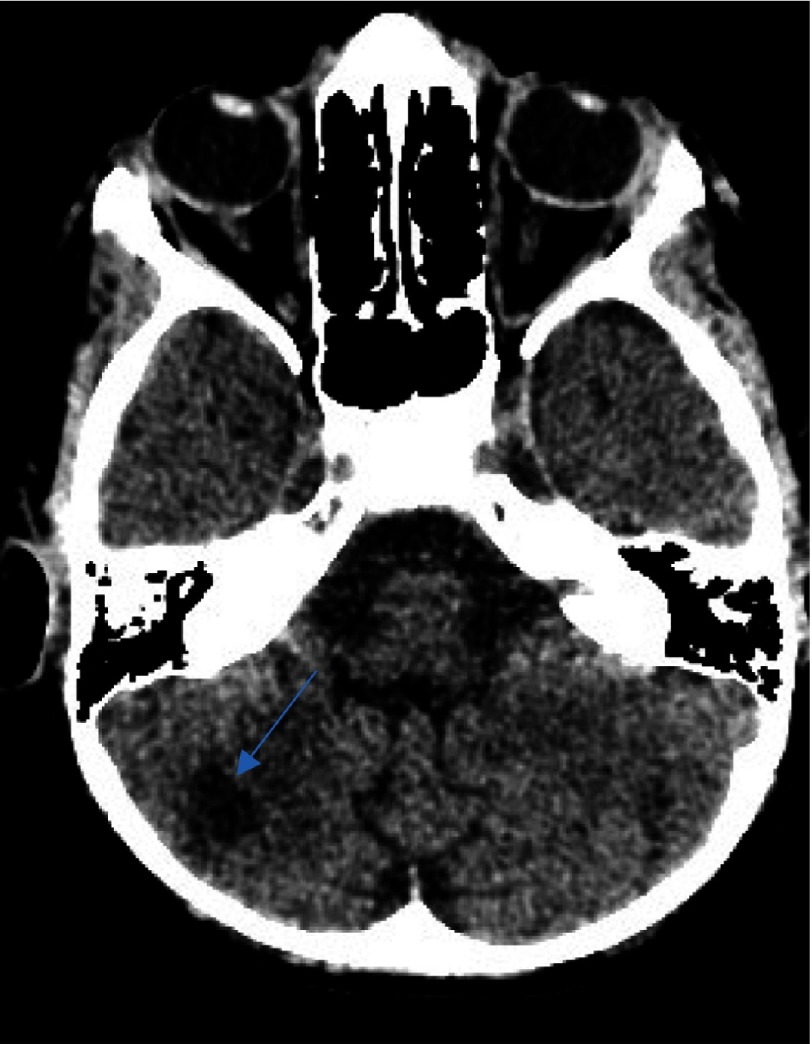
CT scan after the second ischemic insult. Note the hypointensity signal area at the depth of the right cerebellar hemisphere (arrow).
